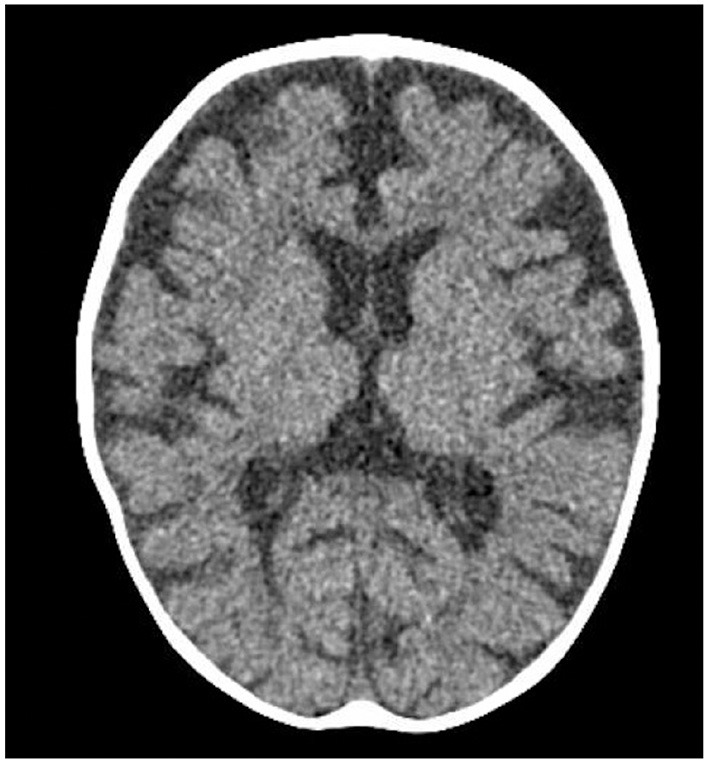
Axial head CT image showing marked cerebral atrophy with reduced white matter volume.
